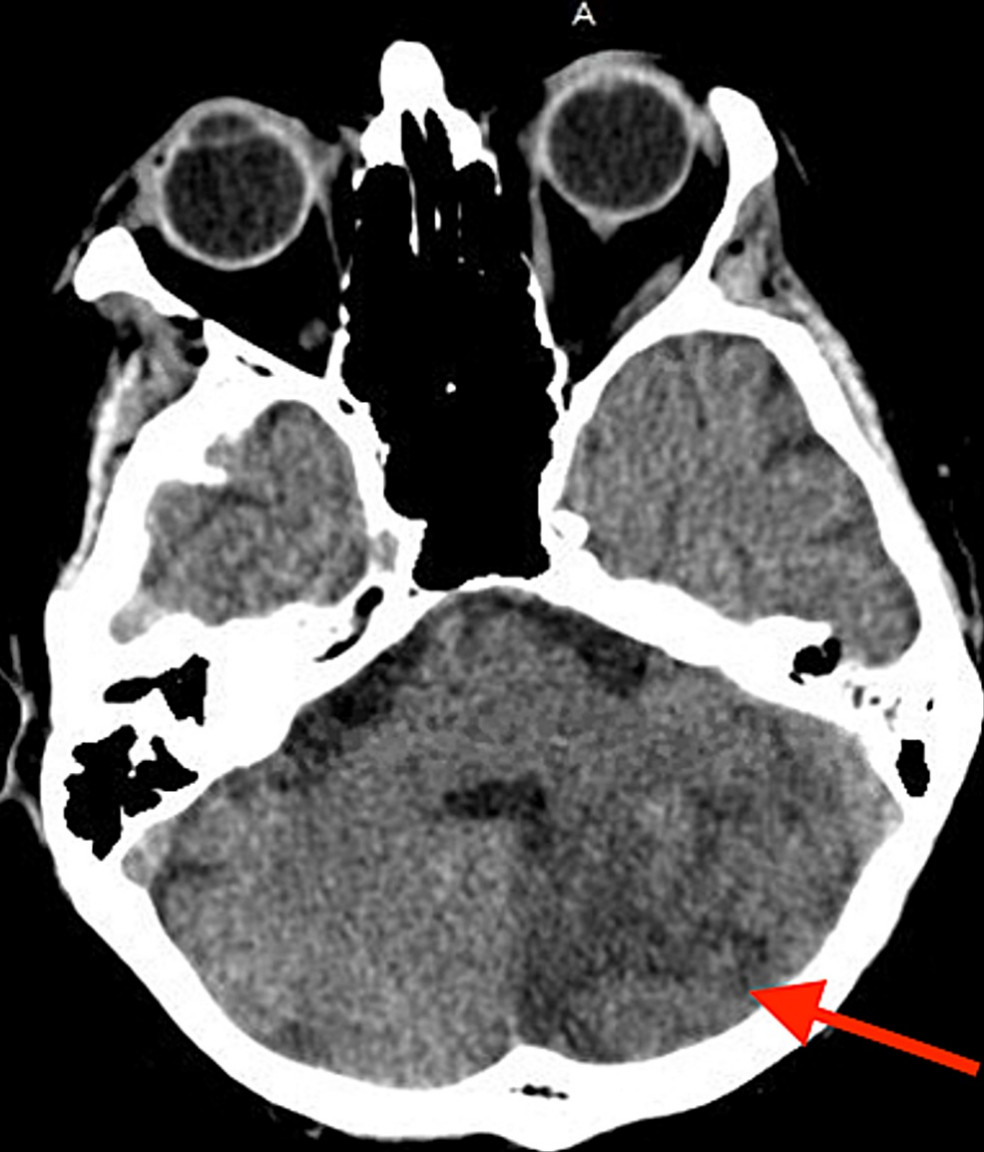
Computed tomography scan of the head without contrast showing an evolution of the left cerebellar infarct with patchy low attenuation in the left cerebellum that appears slightly smaller compared to prior CT.
